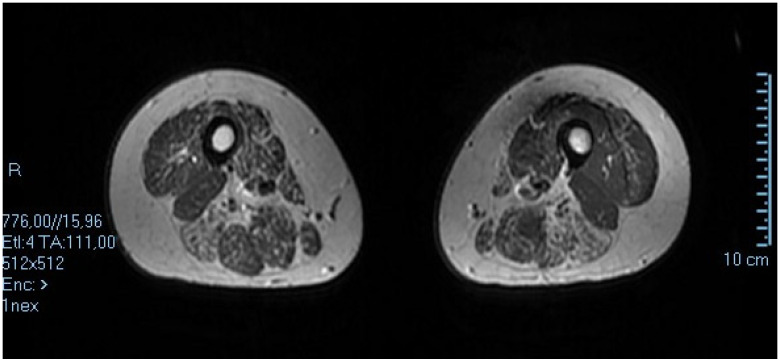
Muscle MRI of proband’s thigh at age 35 shows asymmetric involvement of both anterior vastus lateralis and posterior semitendinosus and semimembranosus muscles with evidence of connective tissue replacement.
